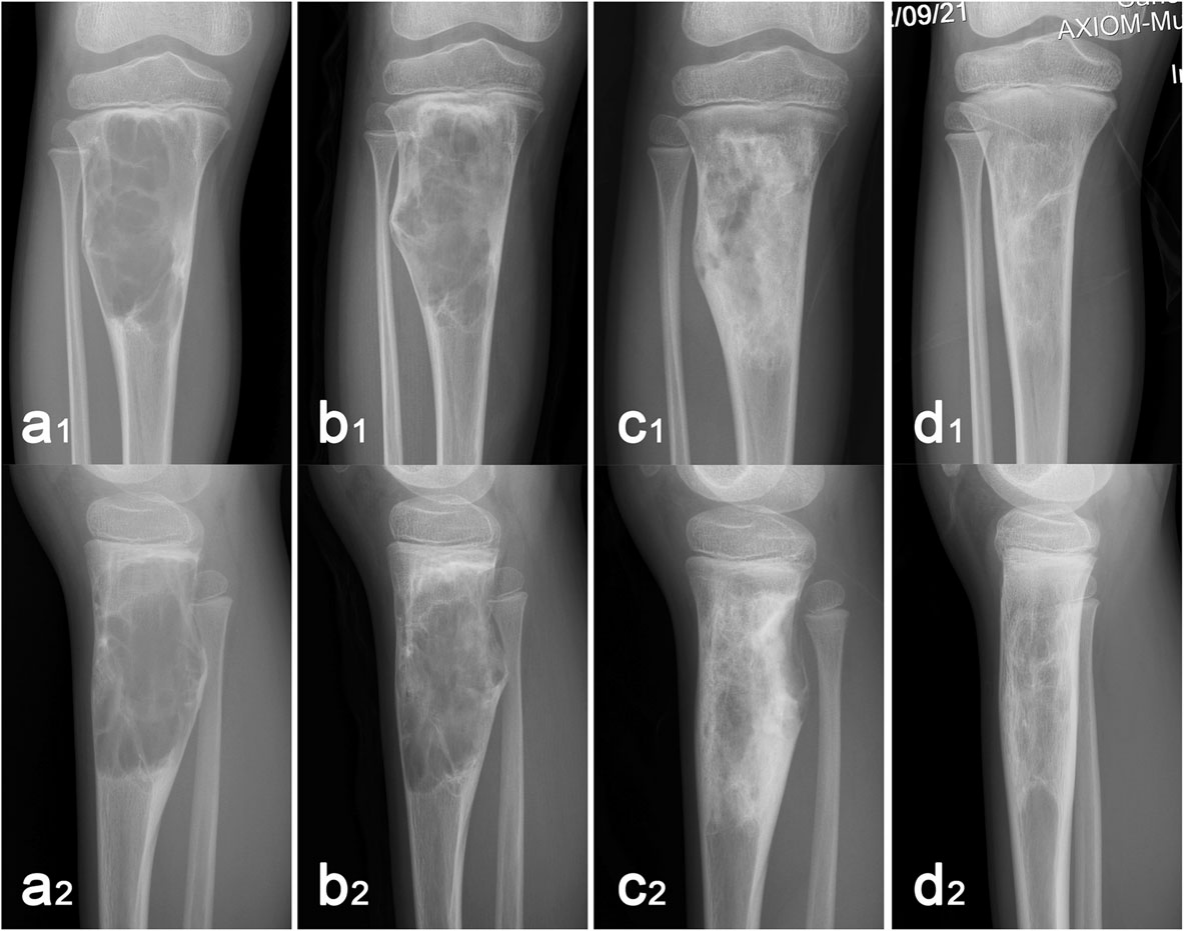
A 10-year-old boy treated with Intracystic methylprednisolone injection, percutaneous curettage, and autogenous bone marrow grafting. These radiographs show changes in the cyst after one treatment as follow-up time prolongated (1, frontal X-ray; 2, lateral X-ray). a Anteroposterior and lateral X-ray before the treatment. b X-ray at 3 months after the treatment. c X-ray at 6 months after the treatment. d X-ray at 12 months after the treatment. In our series of follow-up results, we found that the cortical bone of the cyst was gradually thickened; the low-density areas within the cyst ossified gradually and eventually reassumed a normal bone morphology
